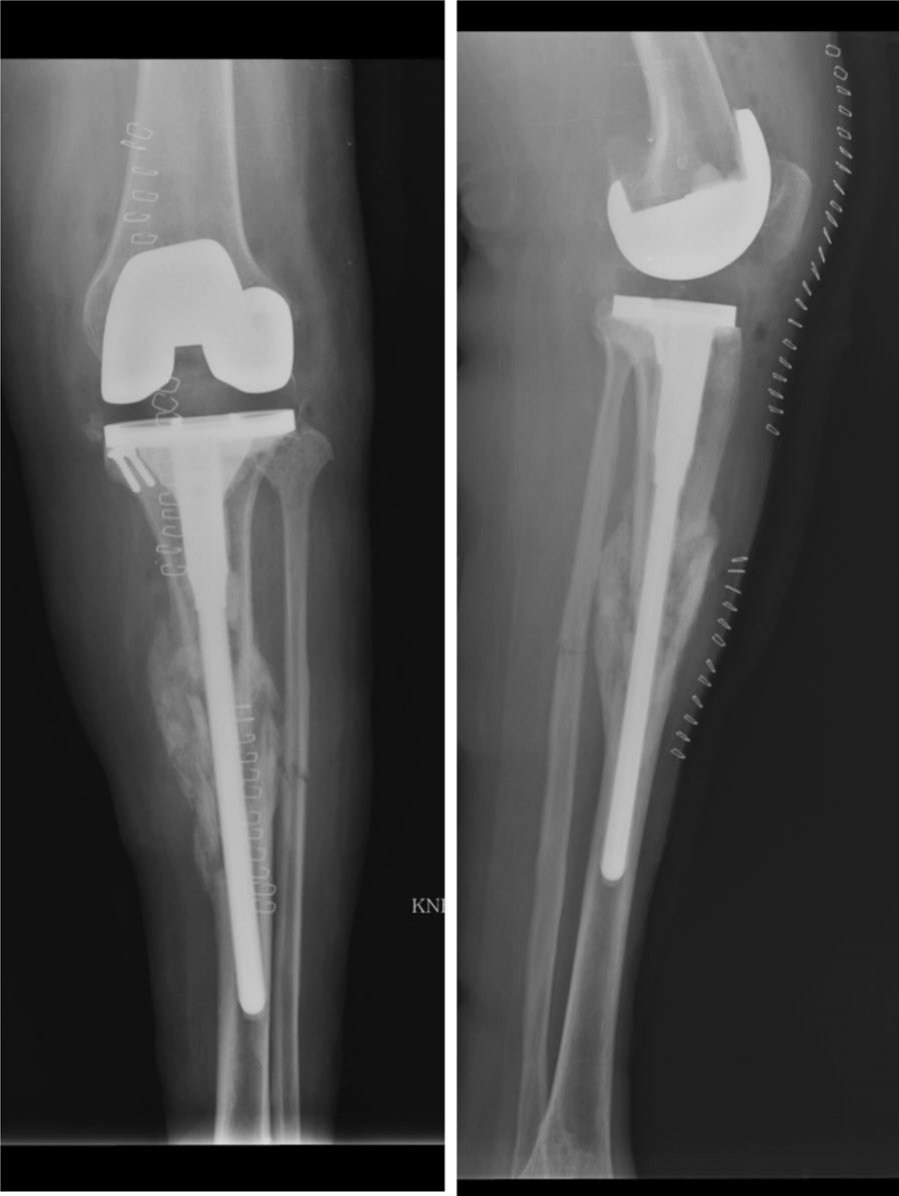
Postoperative radiograph of case 1 patient showing total knee arthroplasty components with long tibial extension rod. The medial plateau fragment was excised and the resultant contained defect was built up with two cortical screws and bone cement. Although the overall postoperative alignment remained as varus, the patient had uneventful recovery during the postoperative period and fracture healing
